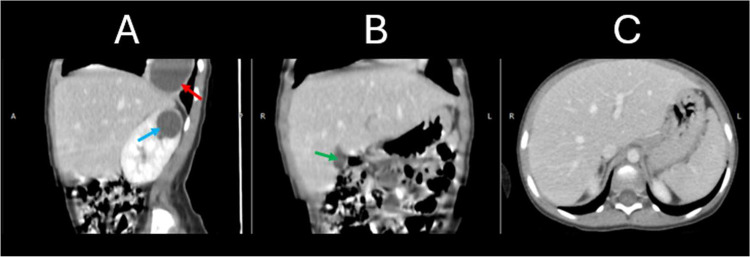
Contrast-enhanced CT scan in the portal venous phase. Multiplanar views including sagittal (A), coronal (B), and axial (C) reconstructions demonstrate no evidence of cystic lesions within the hepatic parenchyma. The gallbladder is visualized with normal morphology (green arrow). A well-defined supra-diaphragmatic cystic lesion is noted in the lower right thoracic cavity (red arrow), and a round, fluid-attenuation cyst is visible in the upper pole of the right kidney (blue arrow).
